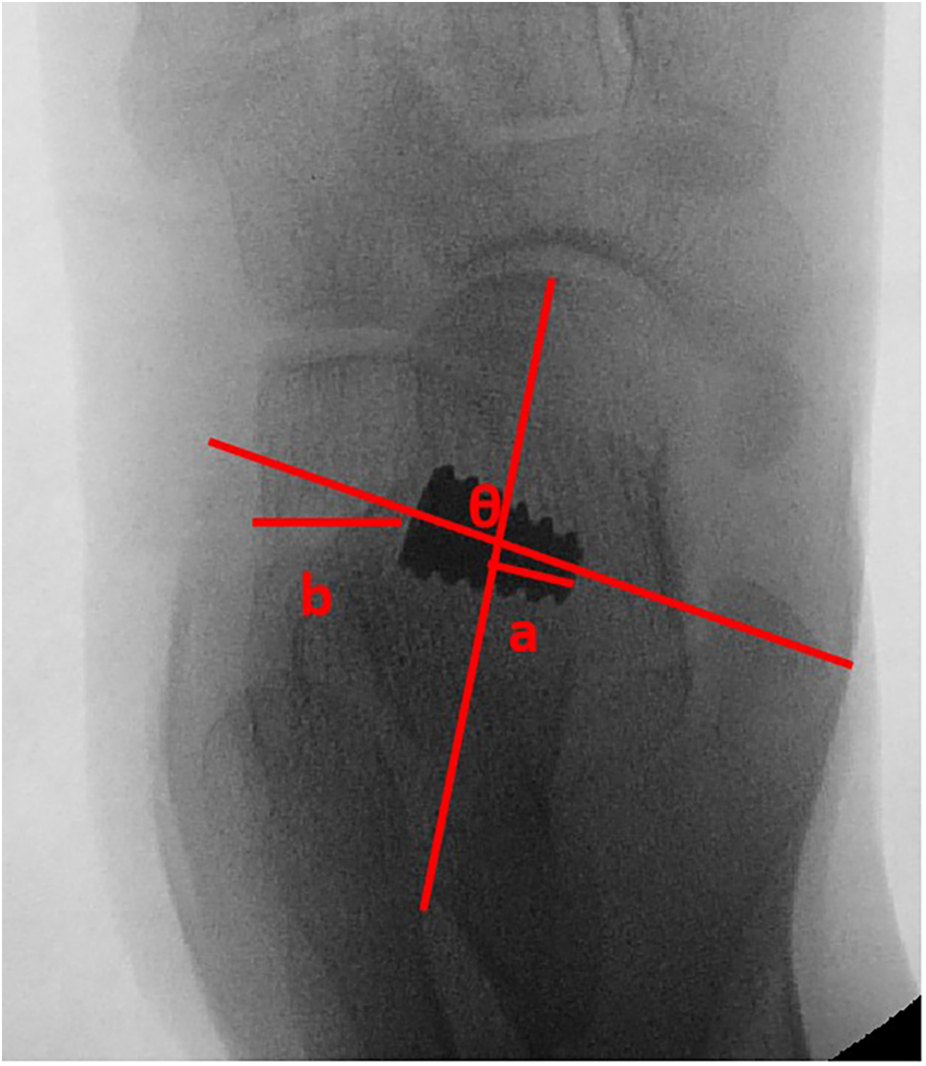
Anteroposterior view of x-ray, demonstrating the position of implant. Angle θ presents the orientation, defined as the angle formed between the talar bisection and the longitudinal axis of implant. Line segment a presents the depth, defined as the perpendicular distance from the medial edge of the implant to the longitudinal talar bisection line. Line segment b presents the distance, defined as the perpendicular distance from the lateral edge of the implant to the lateral calcaneal wall.
